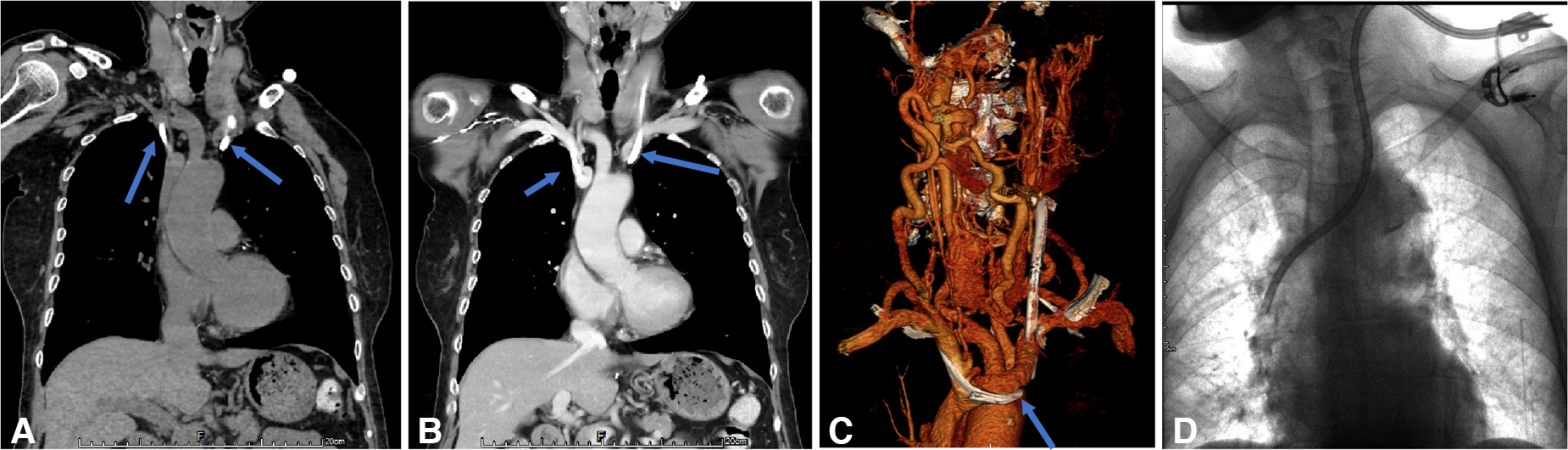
Endoluminal treatment for the catheter malposition into the contralateral innominate vein. (A–C) CT plain and enhancement scan showed the catheter malposition into the right innominate vein from the left side. (D) Exchanging the catheter under fluoroscopy with a guidewire is performed.
